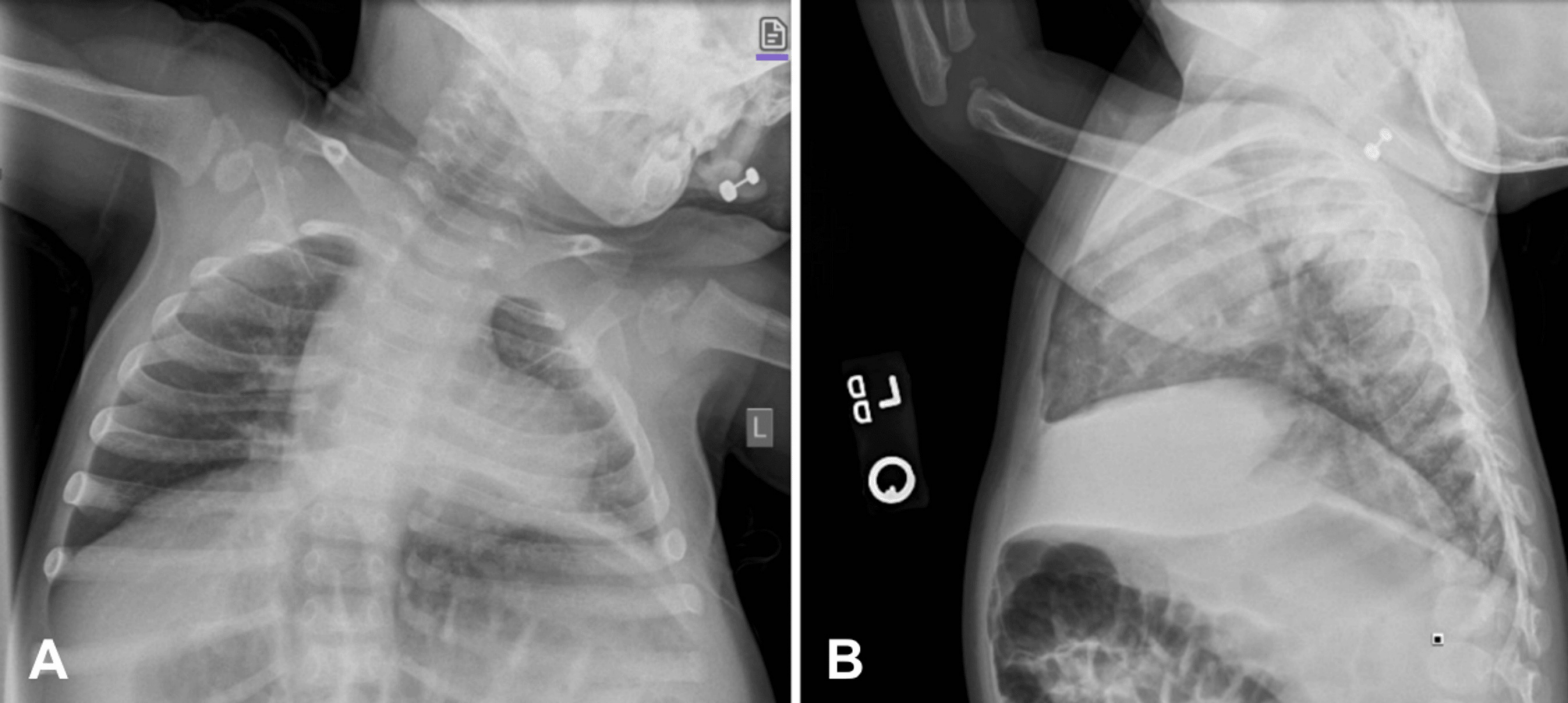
The patient’s chest X-rays with (A) AP and (B) lateral views on presentation were not indicative of signs of pneumonia, croup, or other respiratory disease. The lungs are clear, with no evidence of focal or patchy infiltrates, pleural effusion, or consolidations. No signs of airway narrowing. AP: anteroposterior.
